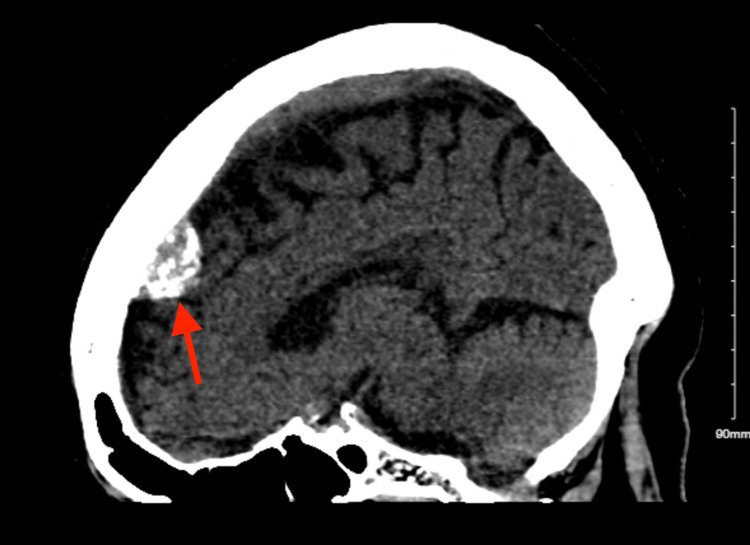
CT scan revealing a meningioma The red arrow points to the meningioma. CT: computed tomography
